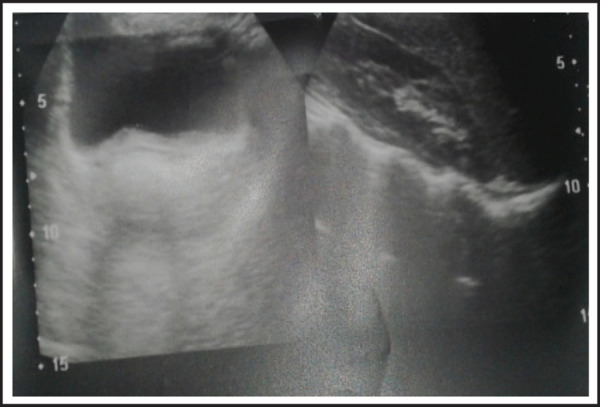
Ultrasound Examination- No Uterus identifieid behind the bladder.
